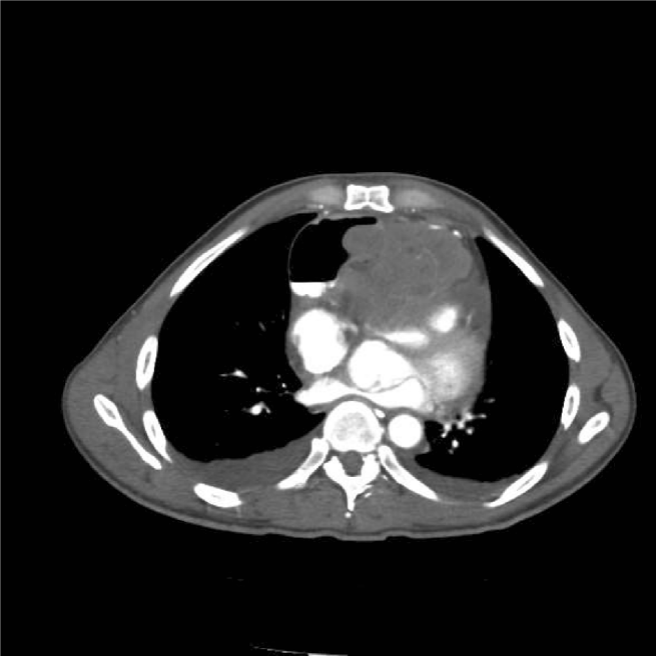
CT scan showing proximity of the mass with the great vessels.
